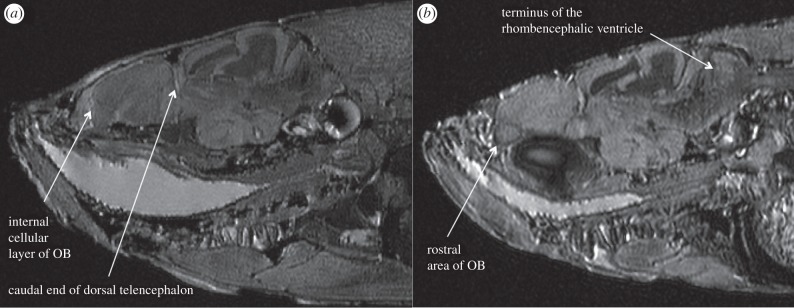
Sagittal magnetic resonance images showing structures used to delineate (*a*) the telencephalon and (*b*) the whole brain. OB, olfactory bulb.
